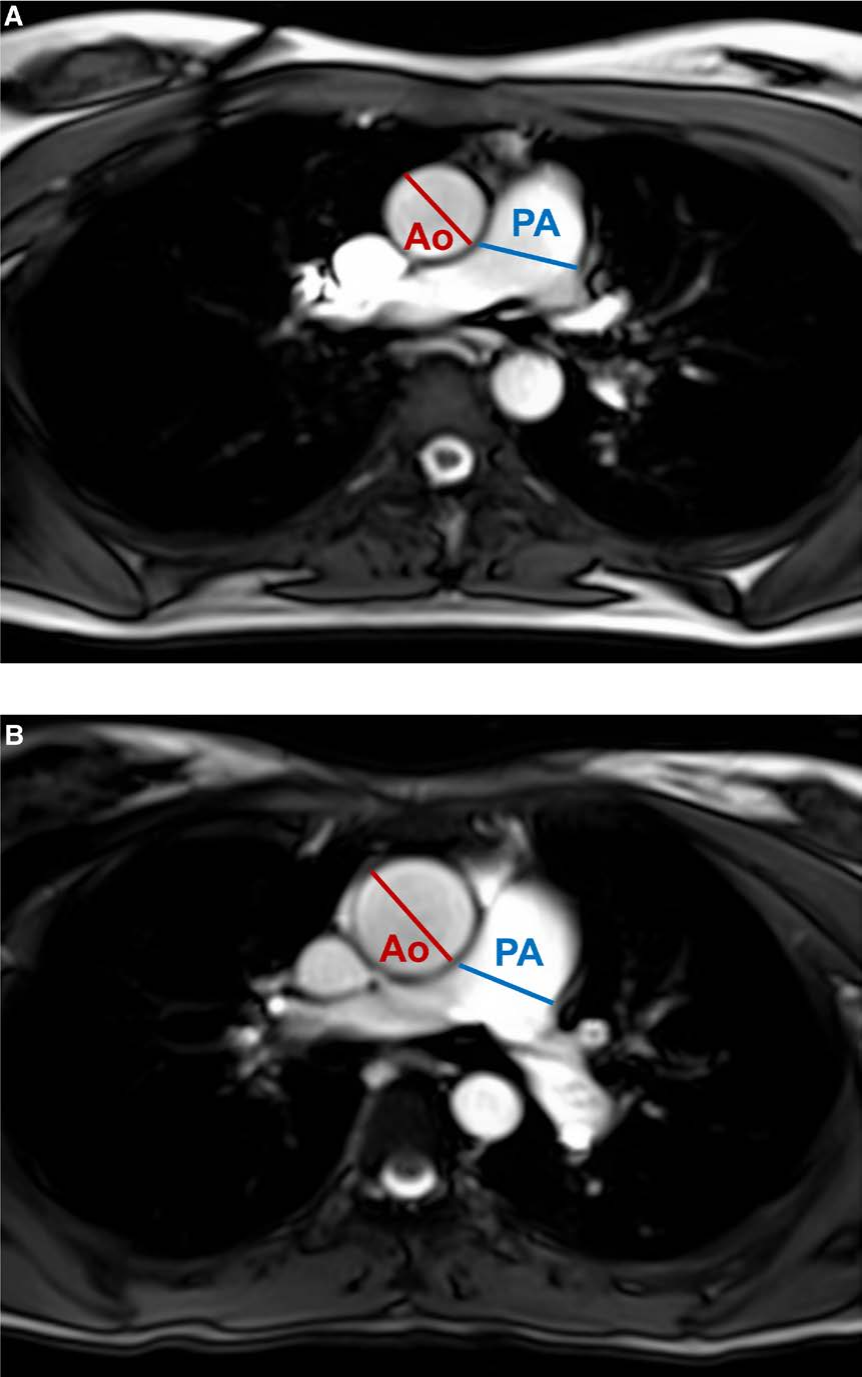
Examples of axial cardiovascular magnetic resonance images at the level of the pulmonary artery (PA) bifurcation from 2 young adult women born at different gestational ages demonstrating increased PA to aorta (Ao) after preterm birth. A 28-y-old woman born at 27^+6^ weeks’ gestation (extremely preterm; PA:Ao is 1.03; A) and a 28-y-old woman born at 40^+2^ weeks’ gestation (term; PA:Ao is 0.79; B).
